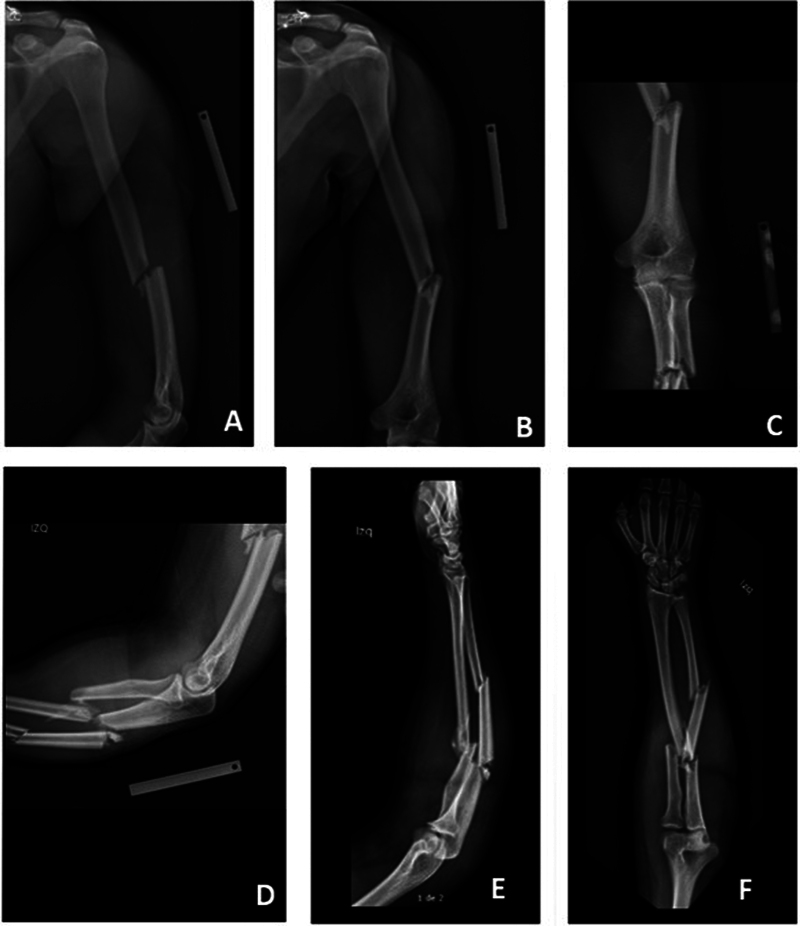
Initial radiological examination of the arm and forearm. The diaphyseal fracture of the humerus is observed in the lateral (A) and anteroposterior (B) planes. X-rays of the elbow showing the relationship between the humerus and forearm fractures in the anteroposterior (C) and lateral (D) planes. Segmental fracture of the ulna and fracture in the proximal third of the radius, in the lateral (E) and anteroposterior (F) planes.
